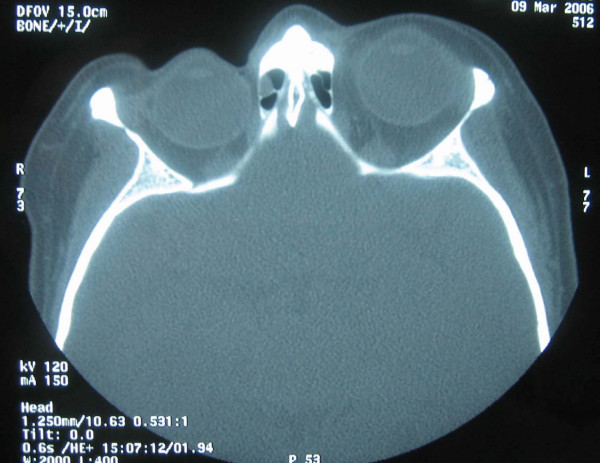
Axial CT scan demonstrating marked right enophthalmos.
